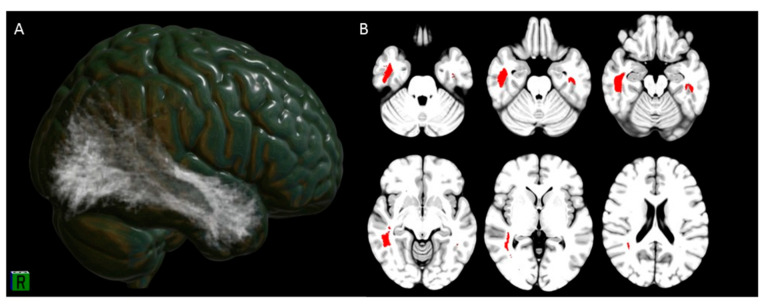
Structural connectivity of regions of hypometabolism in patients with olfactory dysfunction generated through the Brain Connectivity and Behaviour (BCB) toolkit (http://www.toolkit.bcblab.com (accessed on 10 December 2020)), which includes diffusion MRI data from healthy control subjects. Panel (A): The connectome map indicated a significant probability of connection of the hyposmia cluster with the inferior longitudinal fasciculus; Panel (B): tractography results of the hyposmia cluster.
